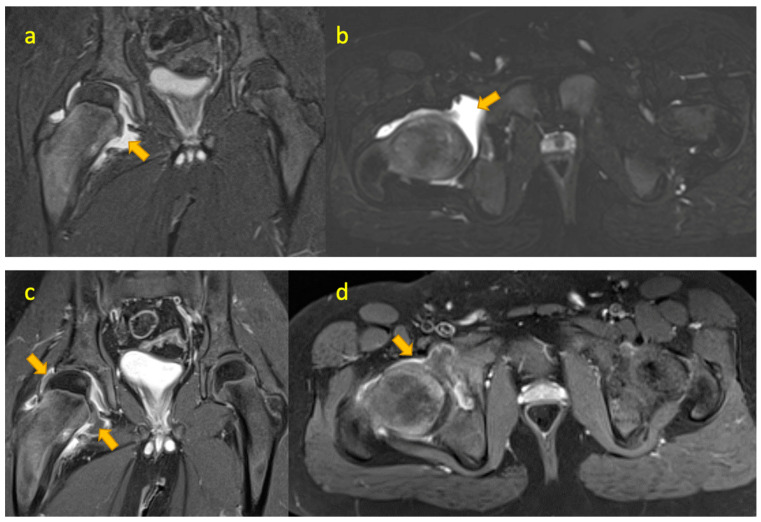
(a–d) MRI hip of a ten-year-old boy who presented with a painful right-sided limp. Coronal STIR, axial T2W FS, and post-gadolinium fat-saturated coronal and axial MRI images showing a large effusion and diffuse synovitis in the right hip proved to be juvenile inflammatory arthritis.
